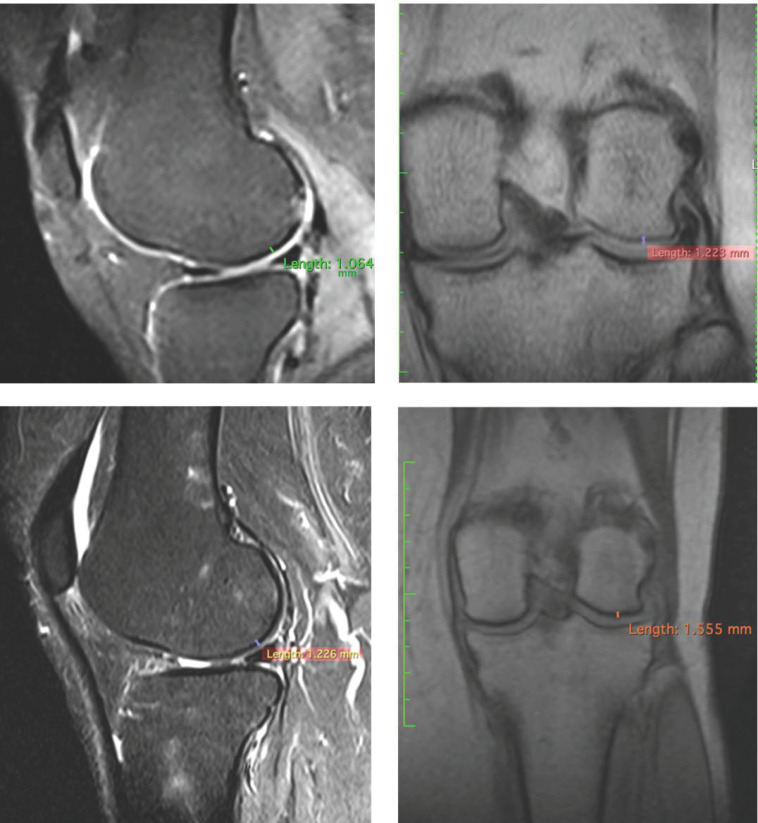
Representative images of MRI of patient no. 1 at 3 years. Images of femoral lateral condyles and measurement of thickness cartilage. We state that different MRI machines and different sequences were used for each patient.
